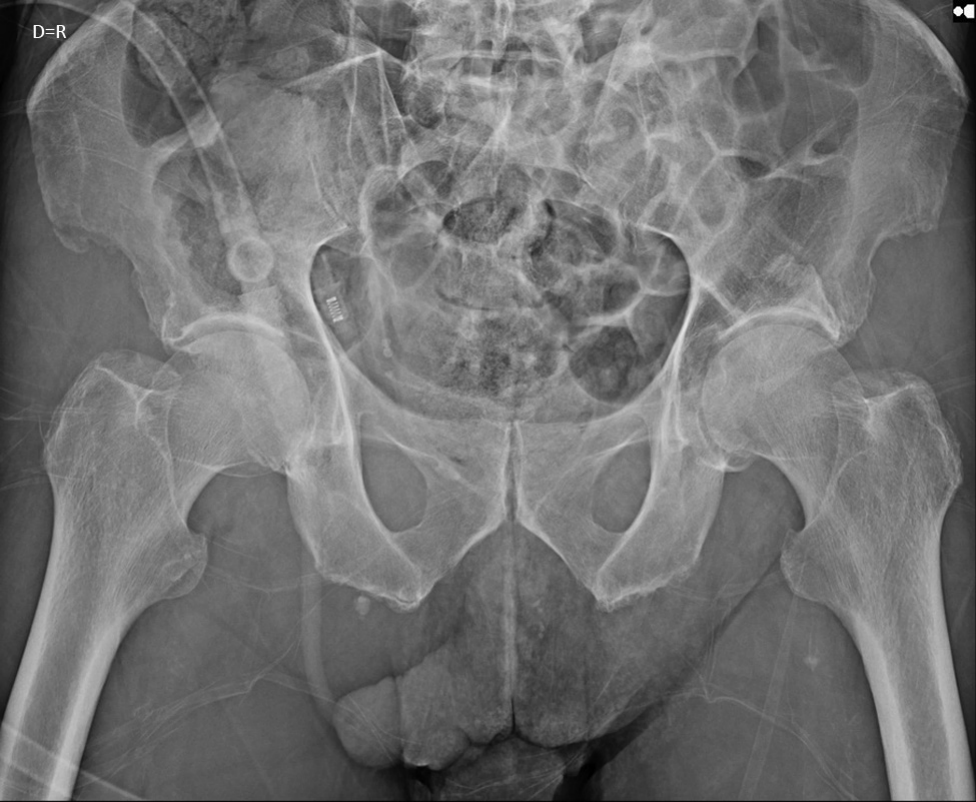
AP radiograph of the pelvis after closed reduction of asymmetric bilateral dislocation of the hips.
